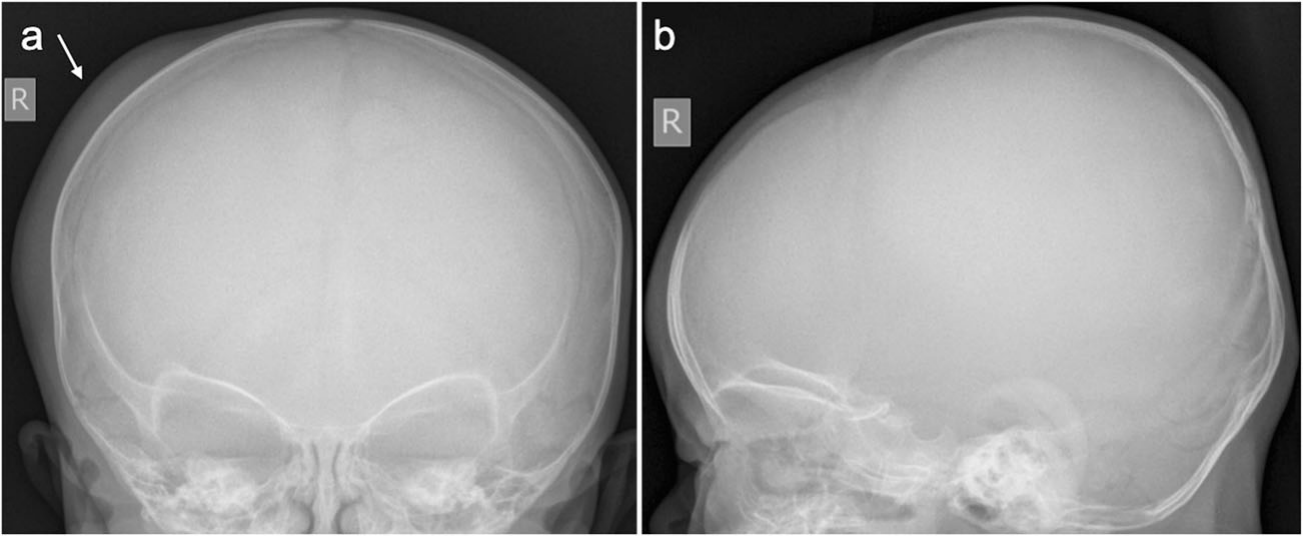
AP (a) and lateral (b) skull radiographs of a 16-week-old infant (case 20) following a reported fall. There is soft tissue swelling over the right side of the head (arrow), but no fracture is identified
